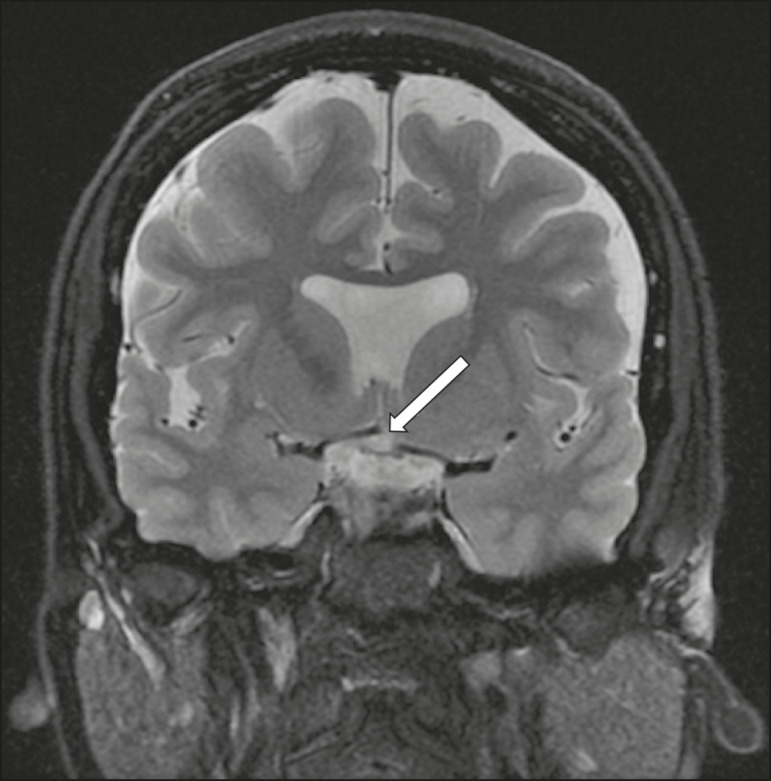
Septo-optic dysplasia. A 30-year-old female with headache and constant dizziness. Coronal T2-weighted MRI scan showing absence of the septum pellucidum and hypoplasia of the optic chiasm (arrow).
